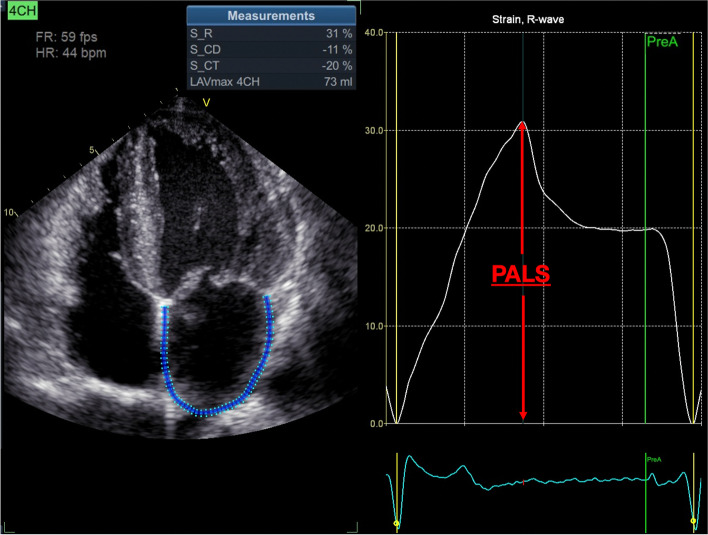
Peak atrial longitudinal strain (PALS). Legend – Measurement of peak atrial longitudinal strain (PALS) using the speckle tracking echocardiography from an apical 4-chamber view in a representative subject
